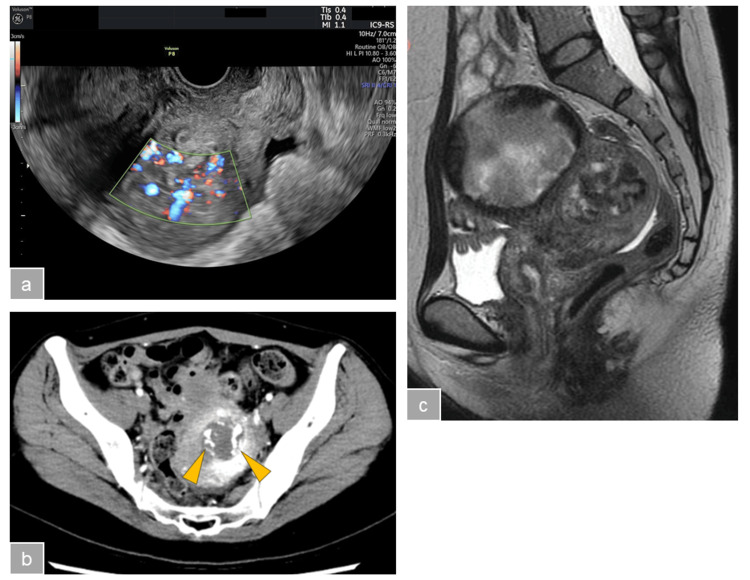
Preoperative imaging A) Transvaginal ultrasonography shows intrauterine mass with prominent blood flow. B) Contrast-enhanced computed tomography (CT) scan shows a hypervascular mass in the uterine cavity that is mainly perfused by the uterine artery (arrowhead). C) T2-weighted magnetic resonance imaging (MRI) scan shows a degenerated myoma in the anterior wall of the uterus, and a 4.5 cm sized heterogeneous mass in the uterine cavity was confirmed.
